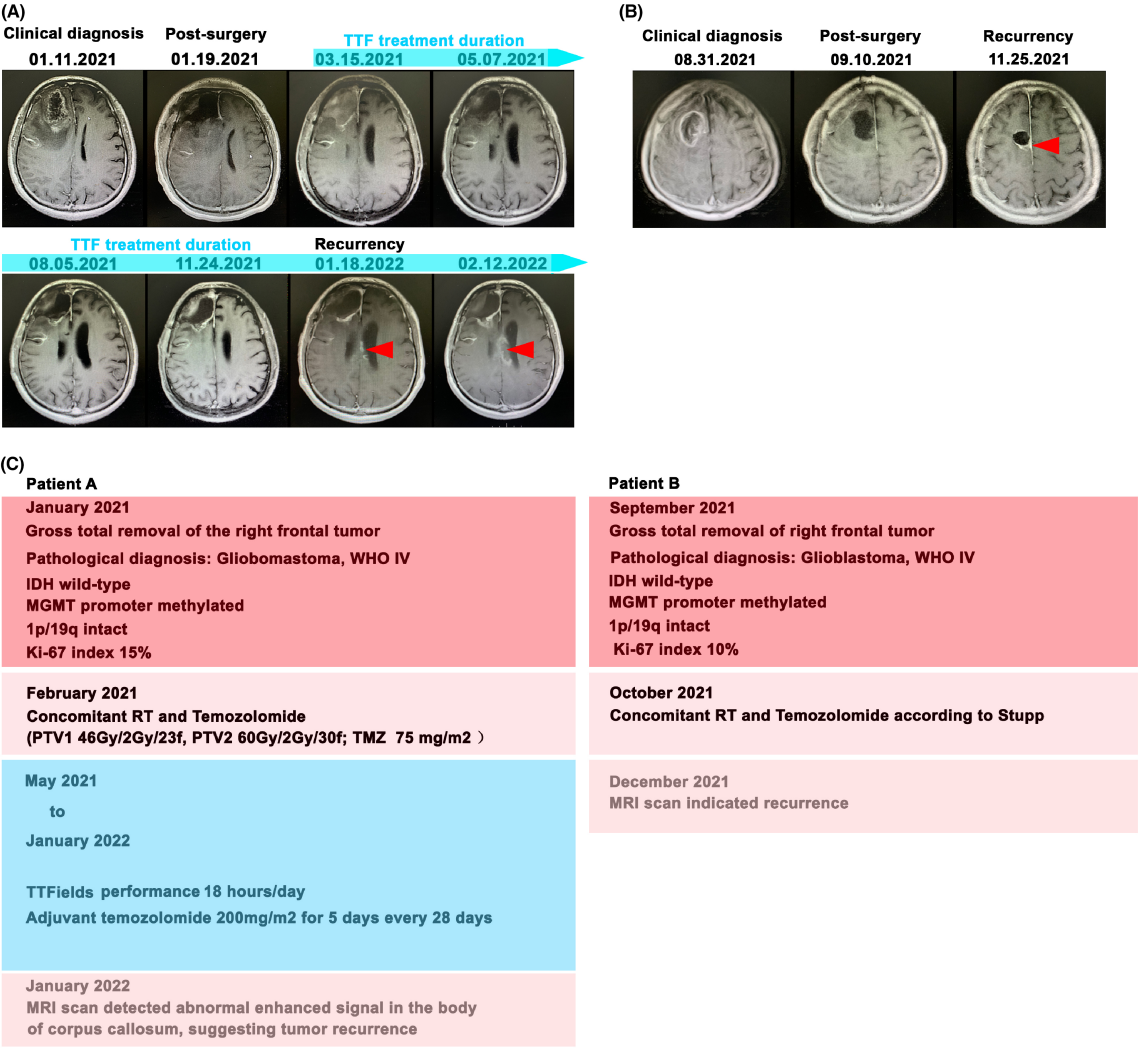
Case study of glioblastoma (GBM) patients who may benefit from TTF. (A) Patient A was initially diagnosed with GBM. “01.11.2021 MRI” indicated right frontal lesion with enhanced signal. Lesion was surgically removed under right frontal craniotomy: “01.19.2021 MRI”. The follow‐up MRI indicated that TT fields therapy was administered 3 months after surgery: “03.15.2021–11.24.2021 MRI”. No recurrence was detected by routine surveillance scans until 9 months after surgery. (B) Patient B was analogously detected the right frontal lesion and diagnosed with GBM. Lesion was surgically removed under right frontal craniotomy: “09.10.2021 MRI”. Unfortunately, recurrency was detected in “11.25.2021 MRI”. (C) Clinical features of patient A and B were demonstrated. Red label: initial diagnosis; Pink label: post‐surgical treatment; Blue label: no recurrency periods; Light pink label: recurrency.
